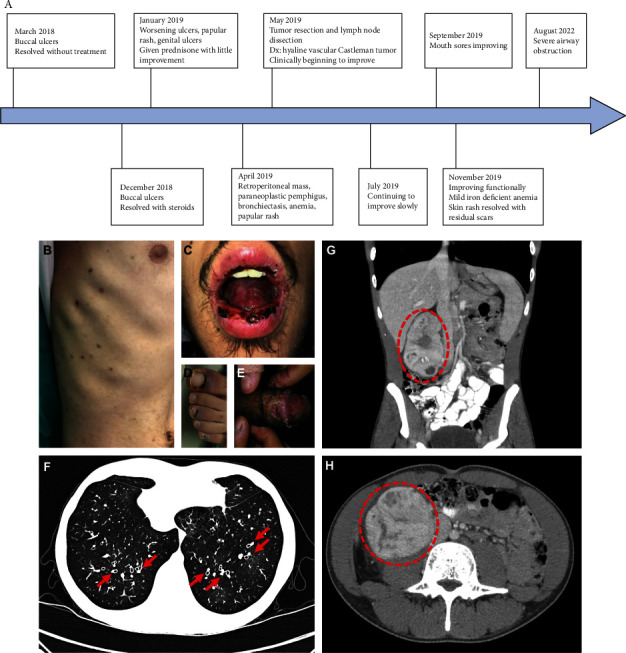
Clinical course and presentation of Castleman disease with retroperitoneal mass: (A) course of patient diagnosis and treatment. (B)–(E) physical findings from April 2019 showing (B) papular rash on the patient's trunk, (C) ulcerative stomatitis, (D) violaceous sanguineous cuticle encrustations, and (E) genital ulcers; (F) chest CT with red arrows indicating signet rings; (G, H) abdominal CT with red dotted circles indicating the retroperitoneal tumor.
